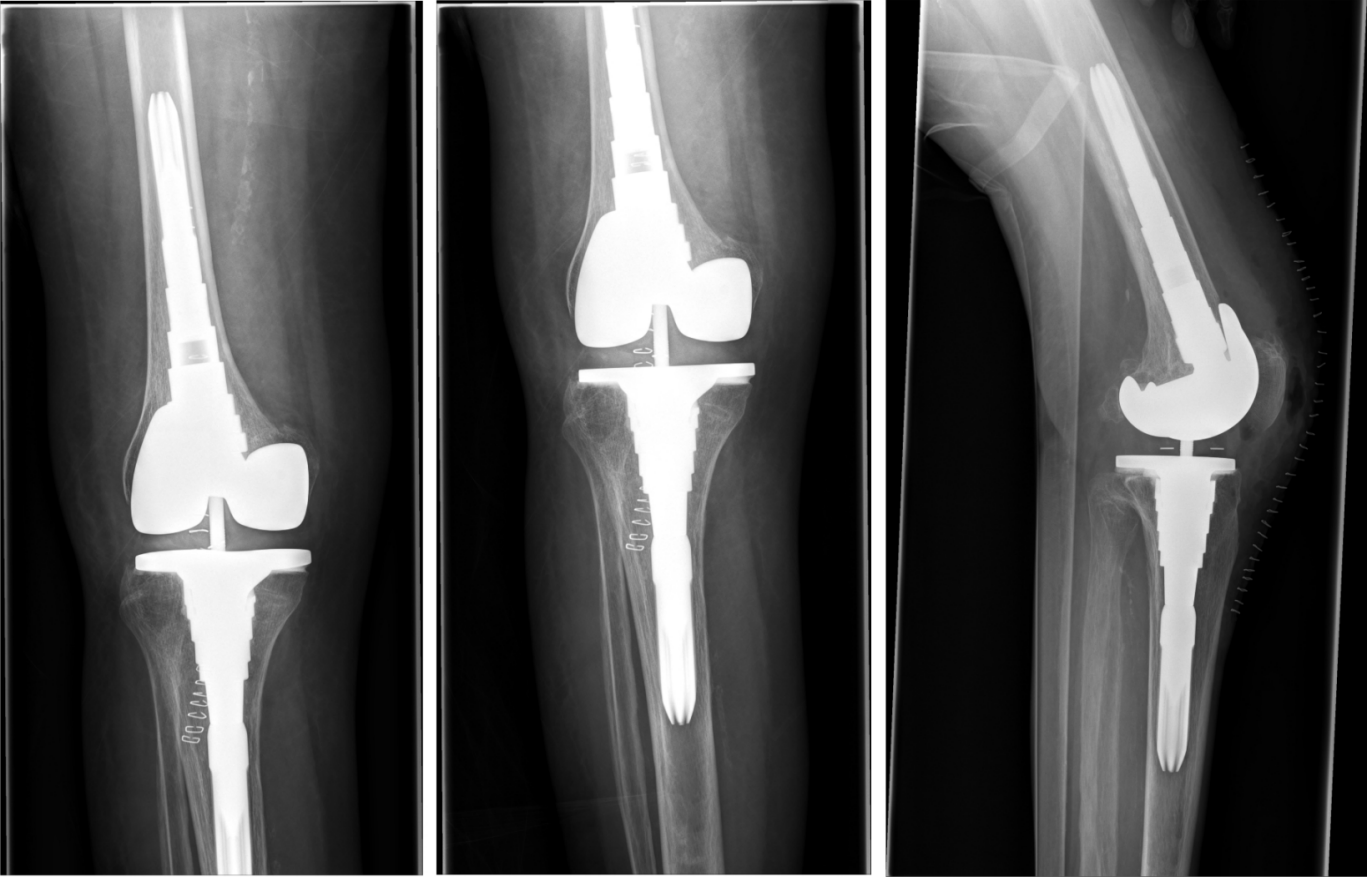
Postoperative x-ray of the right knee joint showing the correct position of the semi-constrained total knee replacement
